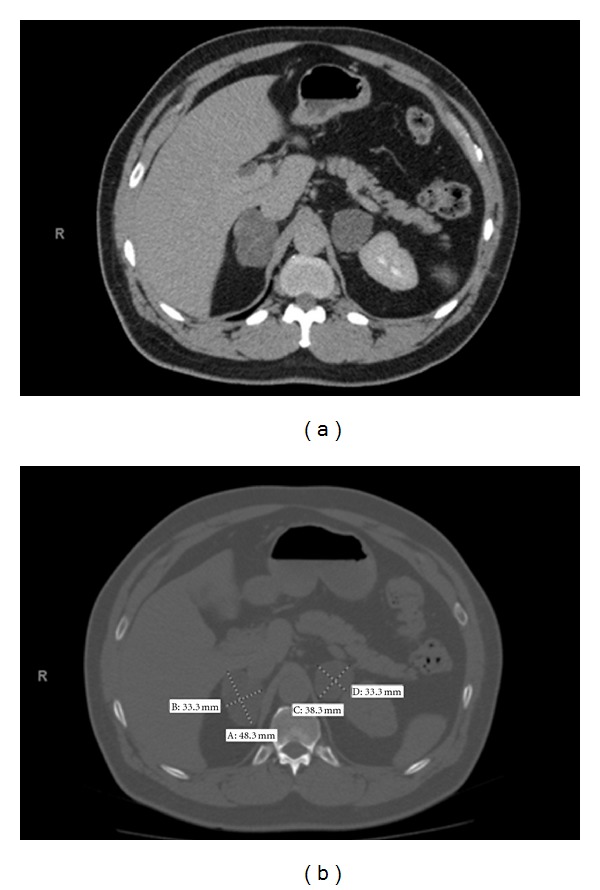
CT scan of abdomen/pelvis with and without contrast as seen during follow-up imaging: lipid-rich adrenal masses bilaterally, right adrenal mass 4.8 × 3.3 cm, previously measured approximately 5.0 × 3.5 cm. Left adrenal mass is 3.8 × 3.3 cm, previously measured 3.7 × 2.9 cm.
